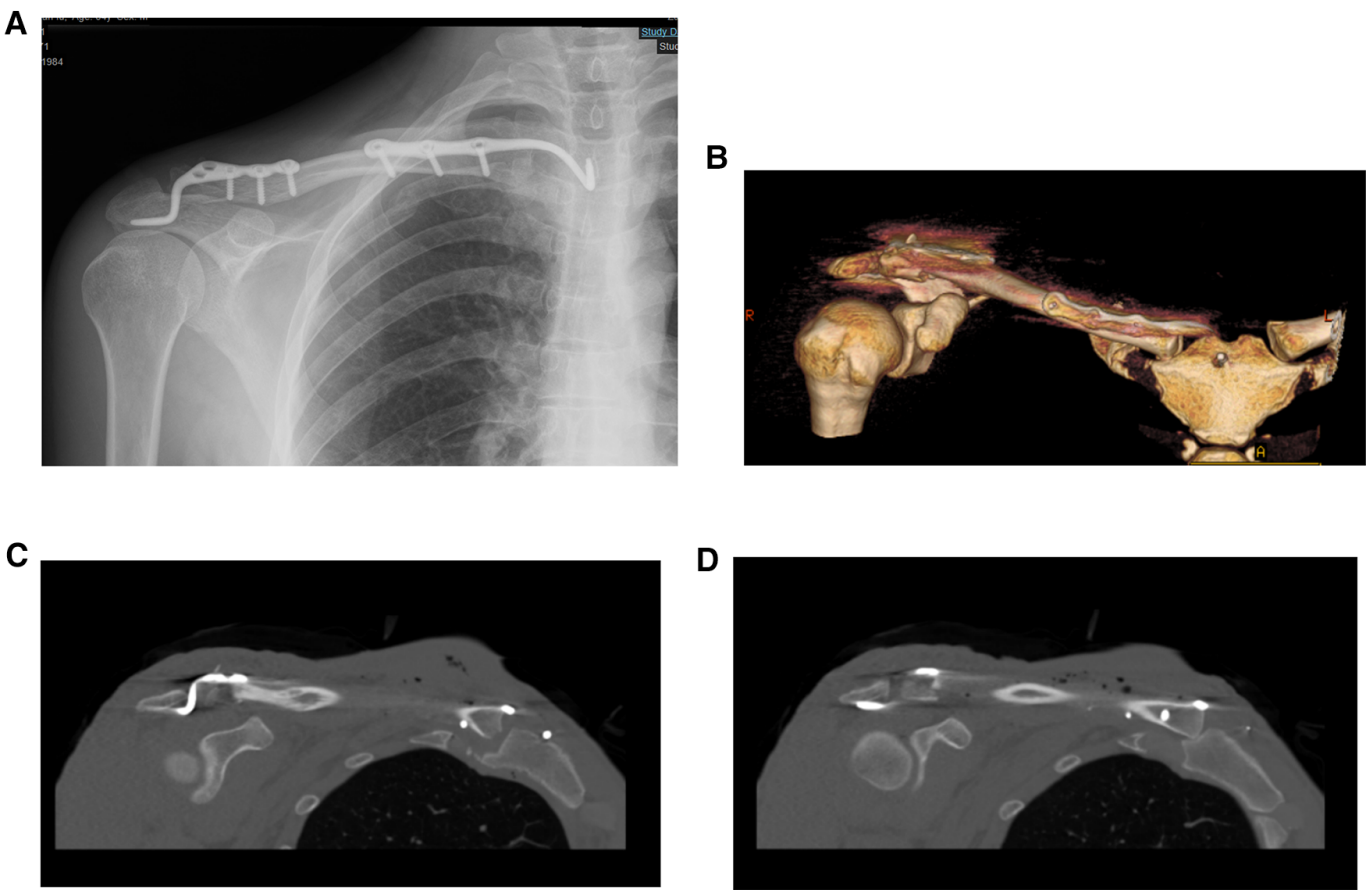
Postoperative imaging of the patient. (A) X-ray image. (B–D) CT images.
